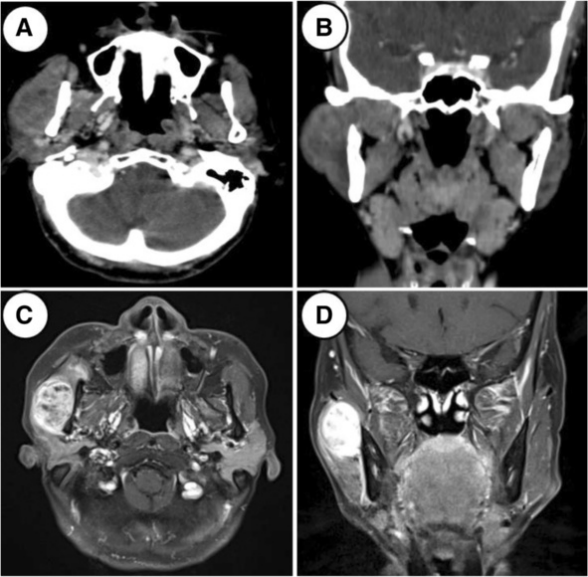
Axial (a) and coronal (b) images of neck computed tomography. The mass shows a heterogeneous density on right parotid gland, with no erosion of adjacent structure. Axial (c) and coronal (d) images of neck magnetic resonance imaging. A 3.5×2.0cm-sized, irregular internal gadolinium-enhanced mass can be seen between the right parotid area extending to masseter muscle. The lesion is relatively well demarcated with smooth margins
